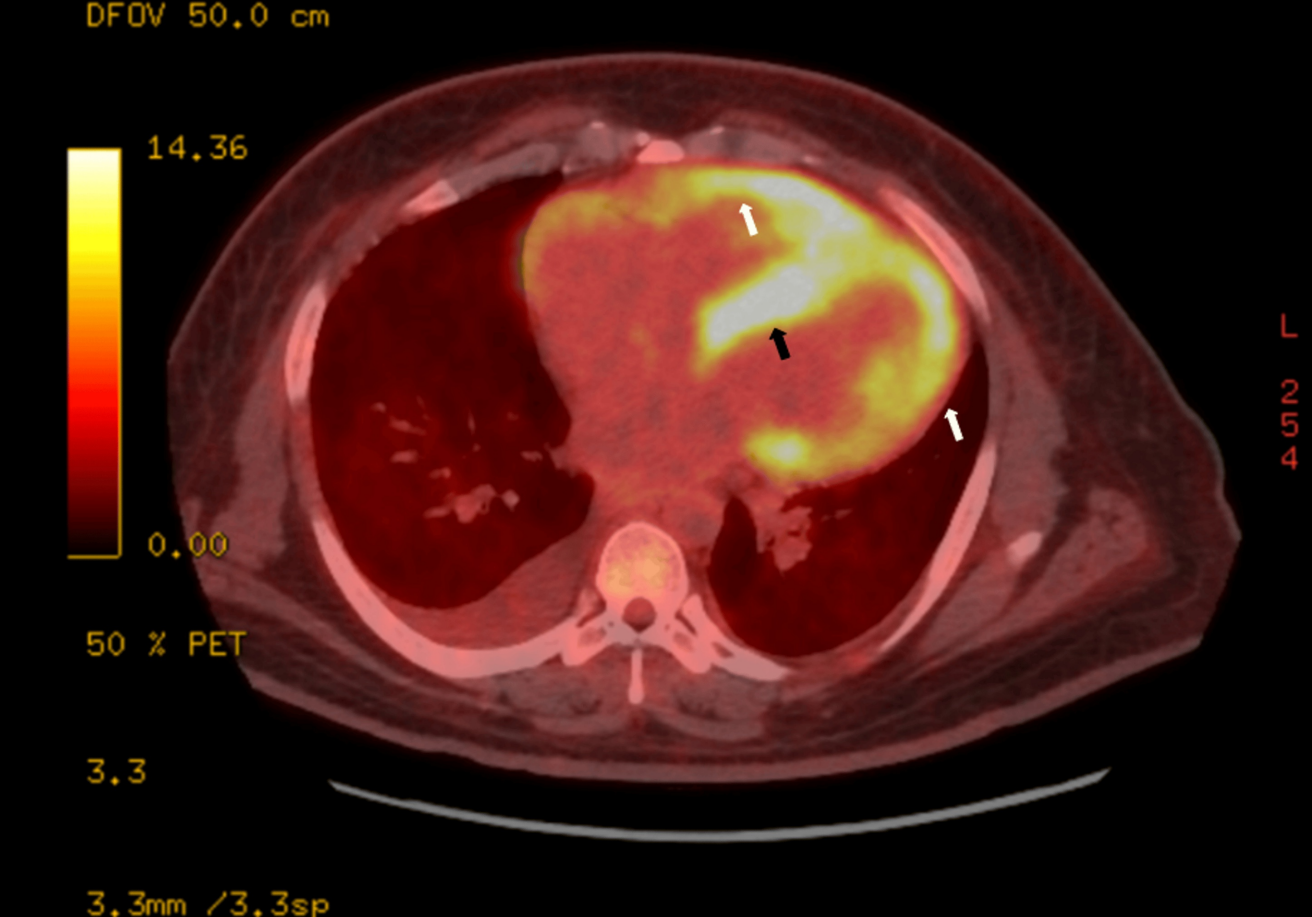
FDG-PET showing diffuse, increased hypermetabolic activity (yellow enhancement) on both ventricles (white arrows), greatest in the interventricular septum (black arrow) FDG-PET: fluorodeoxyglucose-18 positron emission tomography
